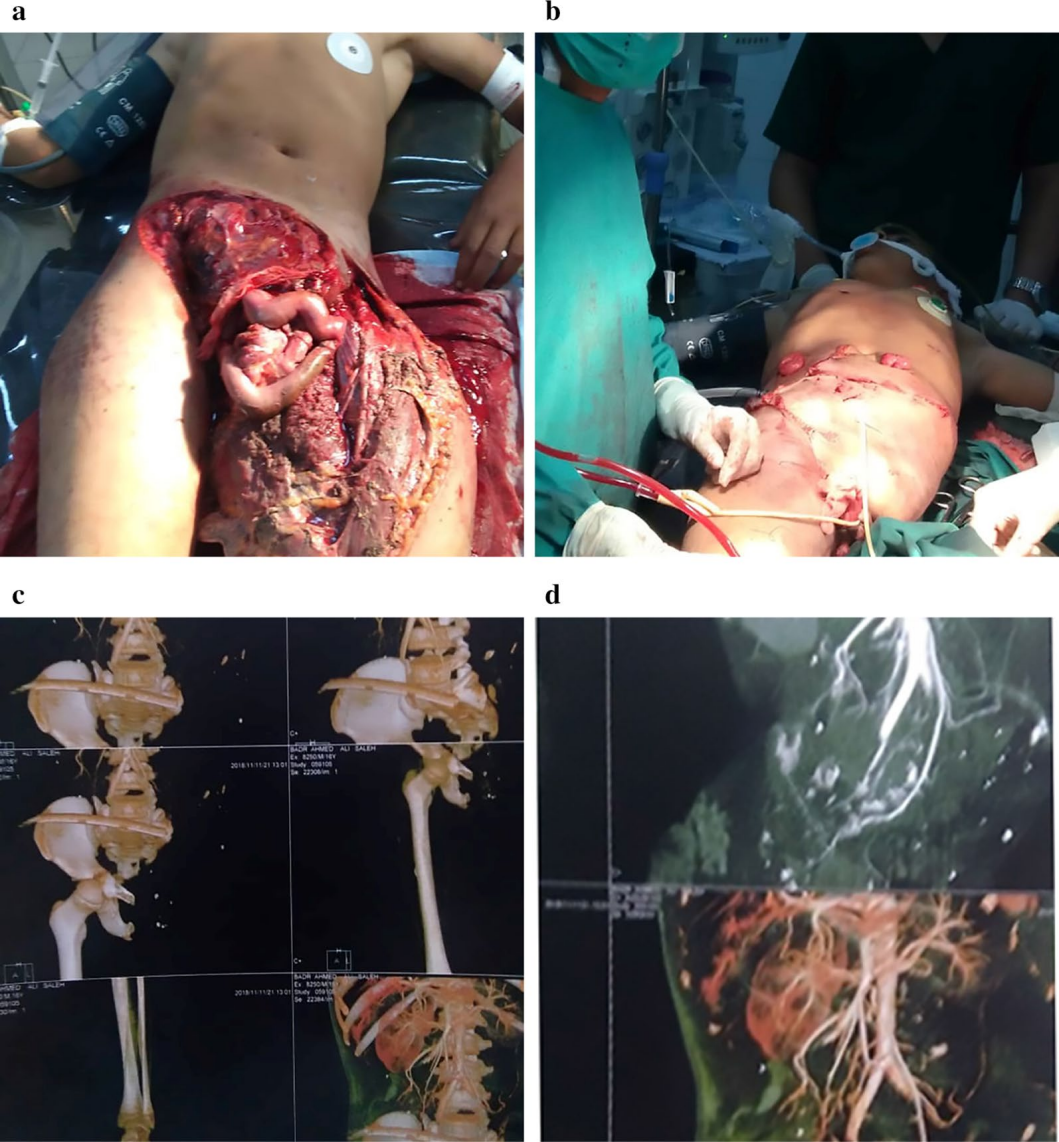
a Details of the wound on the left pelvic side before the initial operation [lower abdomen with exposed urinary bladder, eviscerated bowel, destructed perineum, and almost complete avulsion of the left lower extremity]. b Postoperative clinical situation with left traumatic hemipelvectomy and an ipsilateral anterolateral thigh flap, achieving the primary closure of the wound. c Radiological study showing dislocation of the right sacroiliac joint and symphysis pubis with only some ligamentous attachment. d Computed tomography angiography of the pelvis and right lower extremity indicating the absence of blood flow to the right lower extremity
